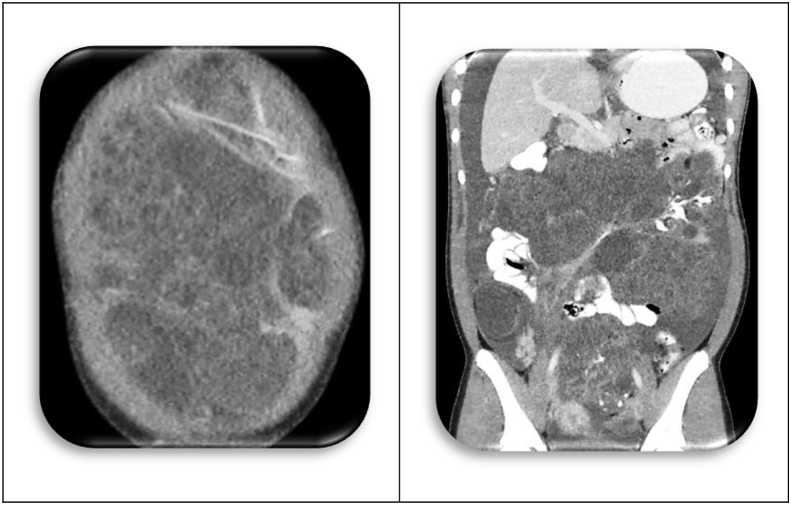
CT scan of Original Tumor.
